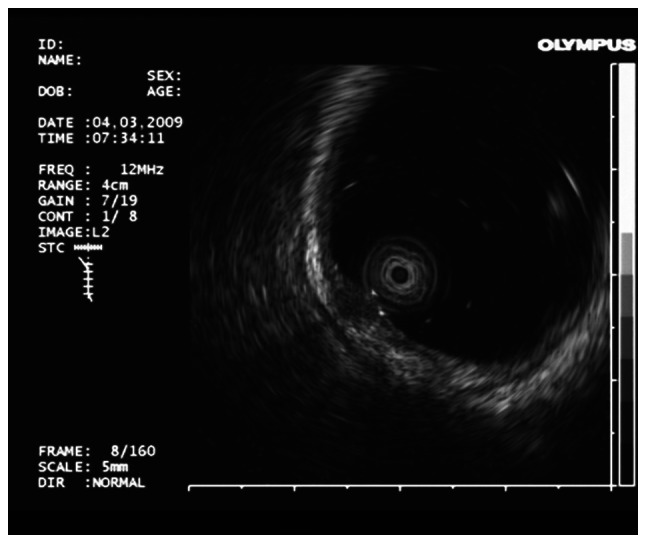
Ultrasonographic examinations still found carcinoid tissue in the in the edge after 2 months.
